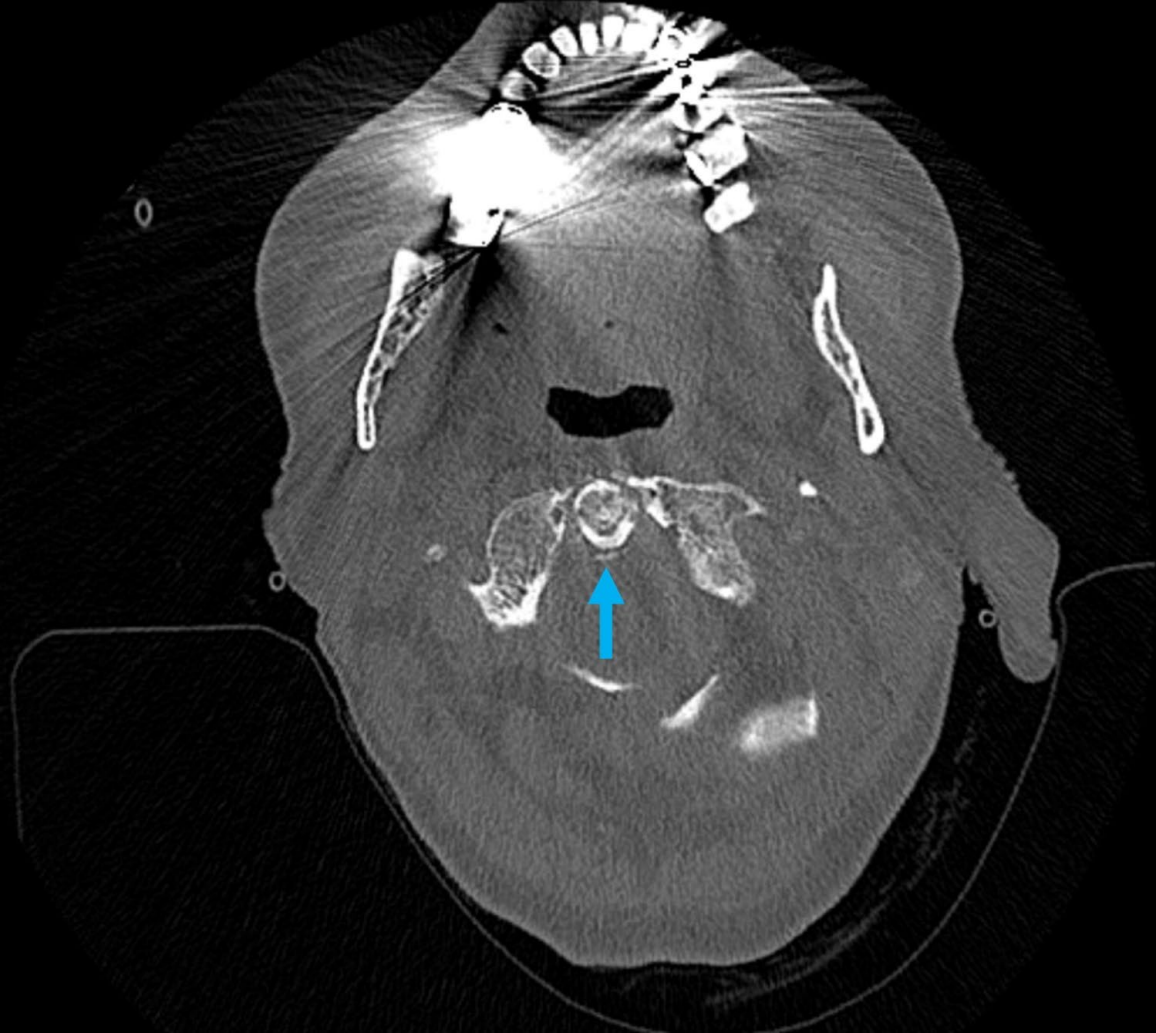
Conventional CT-scan of the cervical spine (axial plane). The arrow indicates typical ligamentous depositions around the odontoid process
